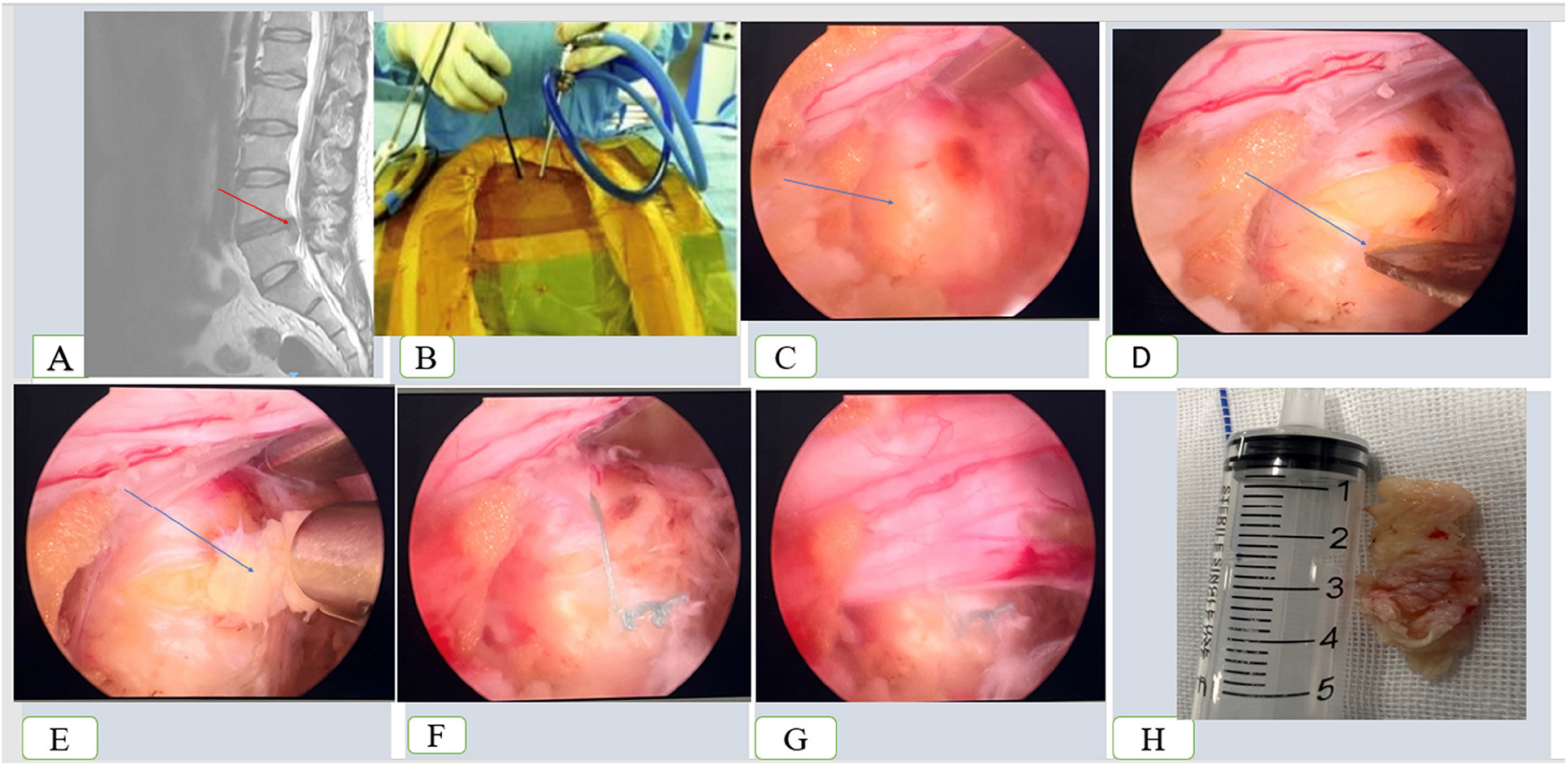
Procedures of the unilateral biportal endoscopic lumbar discectomy on a 54 years female treated for L4/L5 lumbar disc herniation. (A) Preoperative MRI, (B) point of insertion of the endoscopy and the associated instrument, (C) arrow pointing to the herniated disc, (D) process of incision of the herniated disc, (E) process of removing the nucleus fibrosis, (F) sutured annulus ring, (G) released nerve, and (H) nucleus fibrosis.
